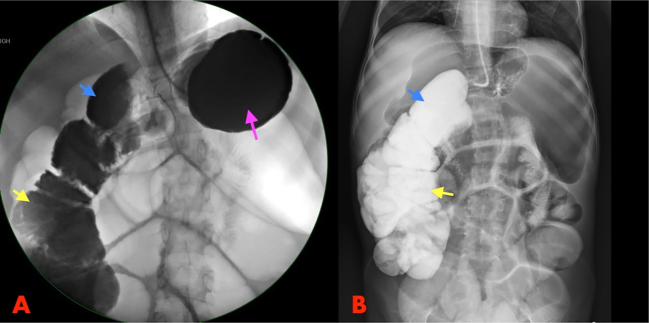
X-ray fluoroscopy of small bowel gastrografin study. (A) The contrast filled the stomach (pink arrow), then directly seen in the hepatic flexure (blue arrow) and ascending colon (yellow arrow). (B) The contrast then moved to the hepatic flexure (blue arrow) and ascending colon (yellow arrow) immediately going from the stomach through the duodenum. The finding is compatible with a fistula between the first part of the duodenum and the hepatic flexure of the colon.
